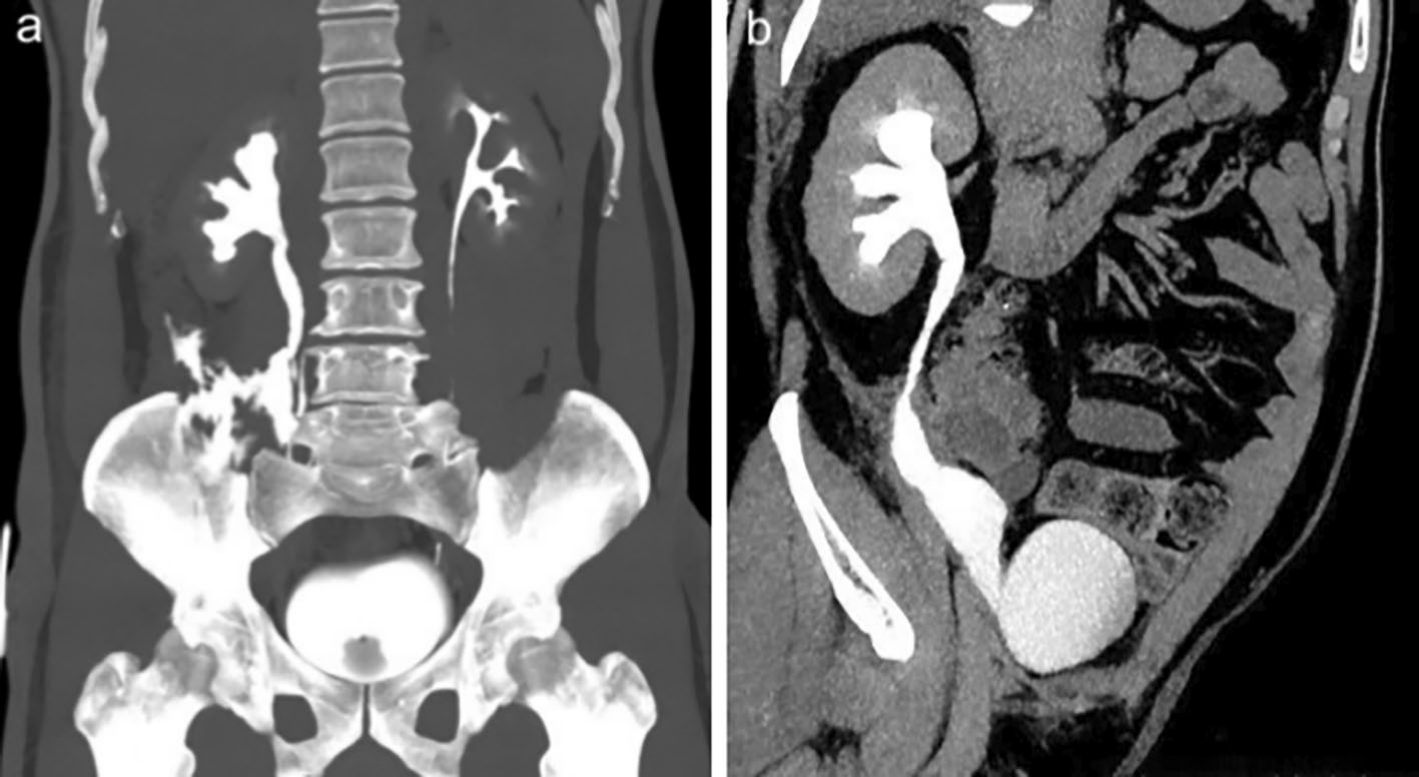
The preoperative and postoperative radiological results. (a) preoperative CT urography; (b) postoperative CT urography.
